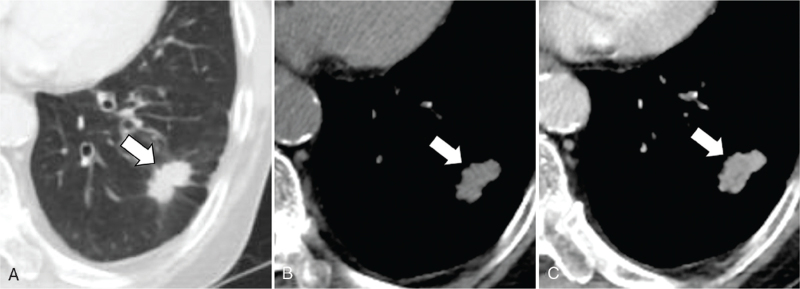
A 78-year-old man with basaloid squamous cell carcinoma of lung. Lung window setting (A), non-enhanced (B), and enhanced (C) CT scans show well-enhancing nodule with spiculated contour (arrow) in left lower lobe periphery. CT = computed tomography.
